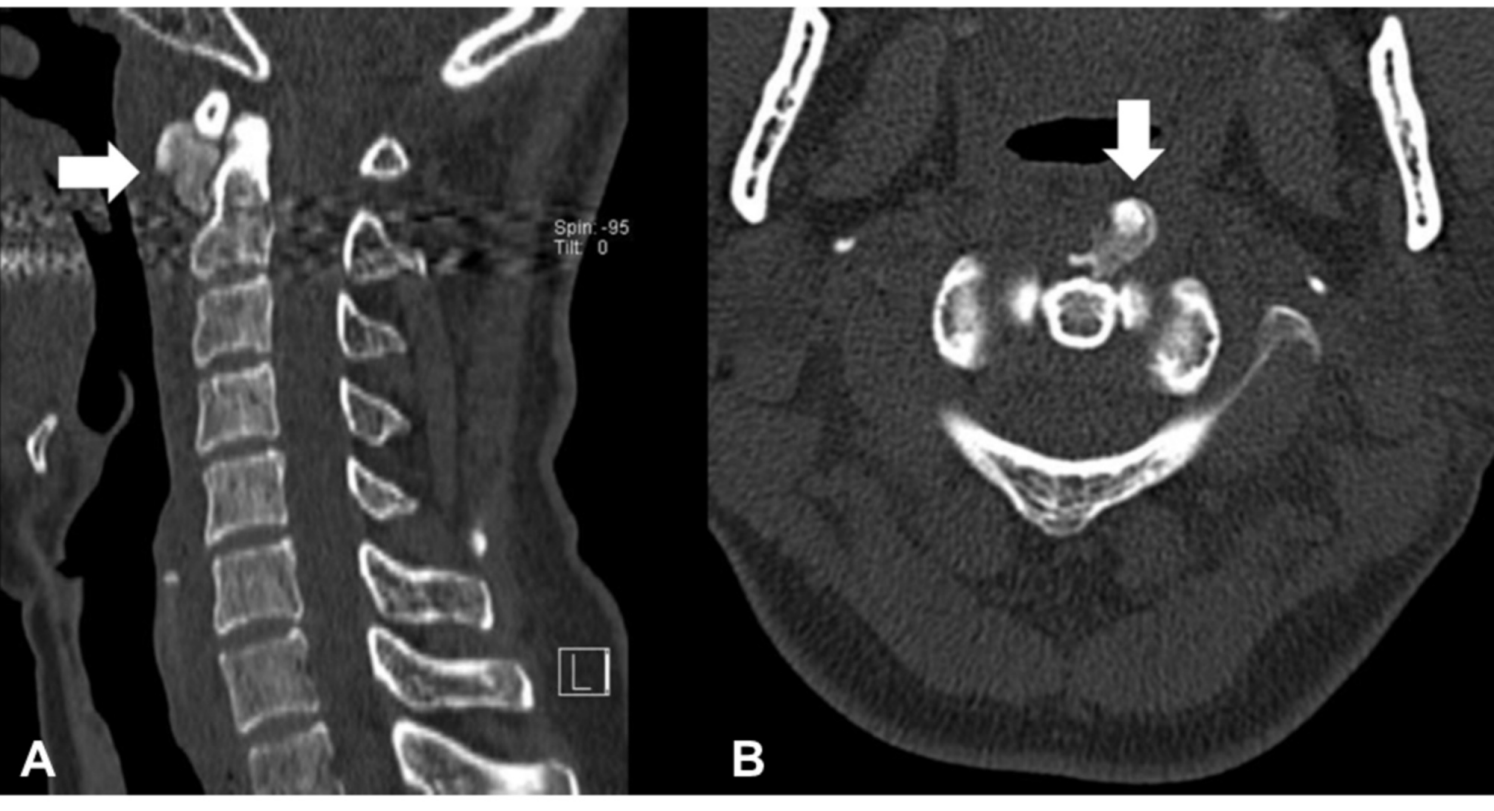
Non-contrast computed tomography (CT) scans of the cervical spine on initial presentation (A) Sagittal and (B) Axial CT scan (bone window) demonstrating amorphous calcification anterior to the C1 and C2 levels with the associated soft tissue swelling (arrows)
